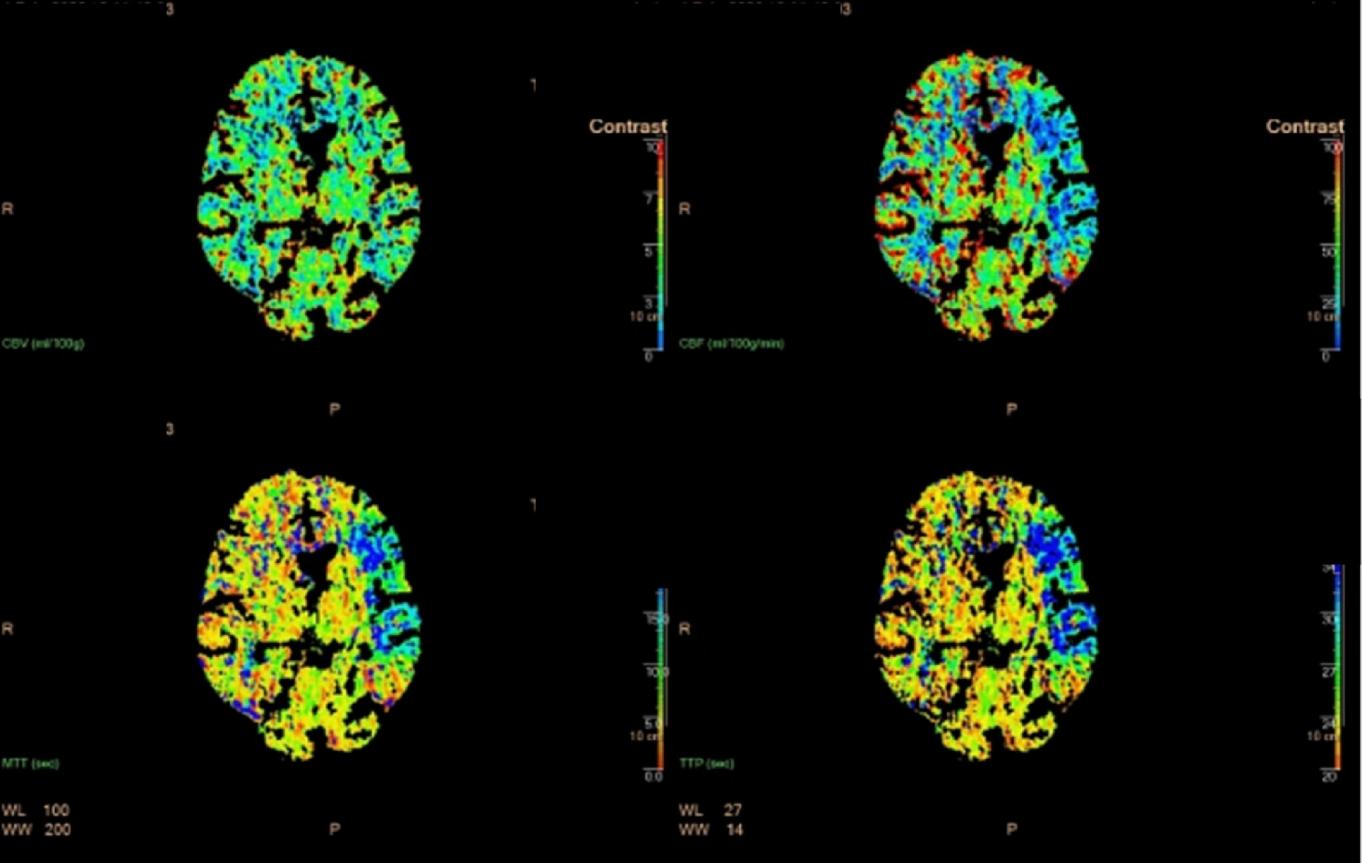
CT perfusion scan demonstrating, starting in the top left and proceeding clockwise: cerebral blood volume (CBV), cerebral blood flow (CBF), time to peak (TTP), and mean transit time (MTT)
